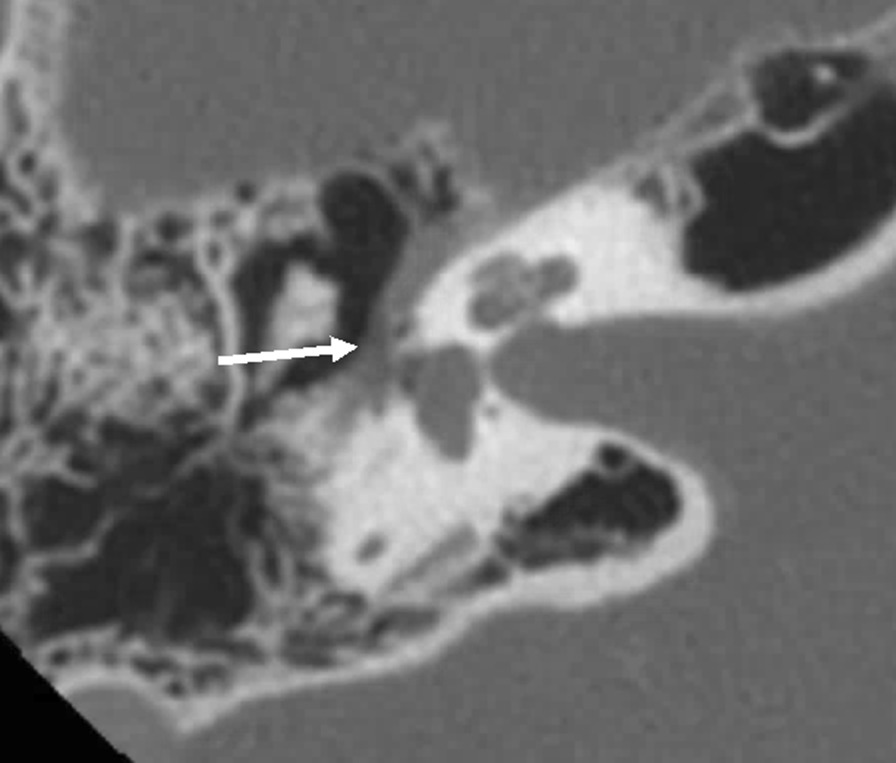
Right-sided temporal bone CT scan. Axial presentation shows dehiscence of the tympanic portion of the right facial nerve (white arrow)
